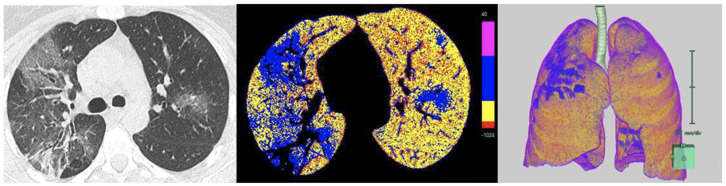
Density mask analysis of CT scan in a 46-year-old female patient. From left to right: axial CT slice with multiple bilateral ground glass opacities, the color image overlays (red = emphysema, yellow = normal parenchyma, blue = ground-glass opacities (GGOs), pink = consolidations) and the 3D volume rendering reconstruction.
